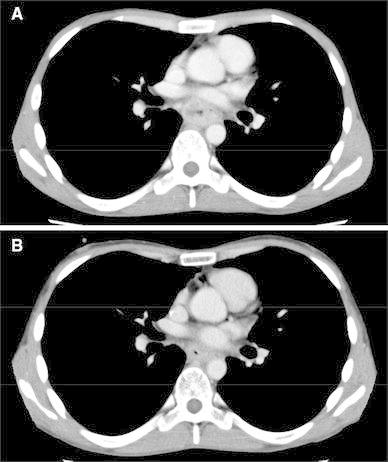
Preoperative radiological images. Computed tomography (CT) scans revealed the tumor invasion of surrounding tissue including aorta prior to initiating neoadjuvant chemoradiotherapy (a). The tumor decreased in size after neoadjuvant chemoradiotherapy (b)
